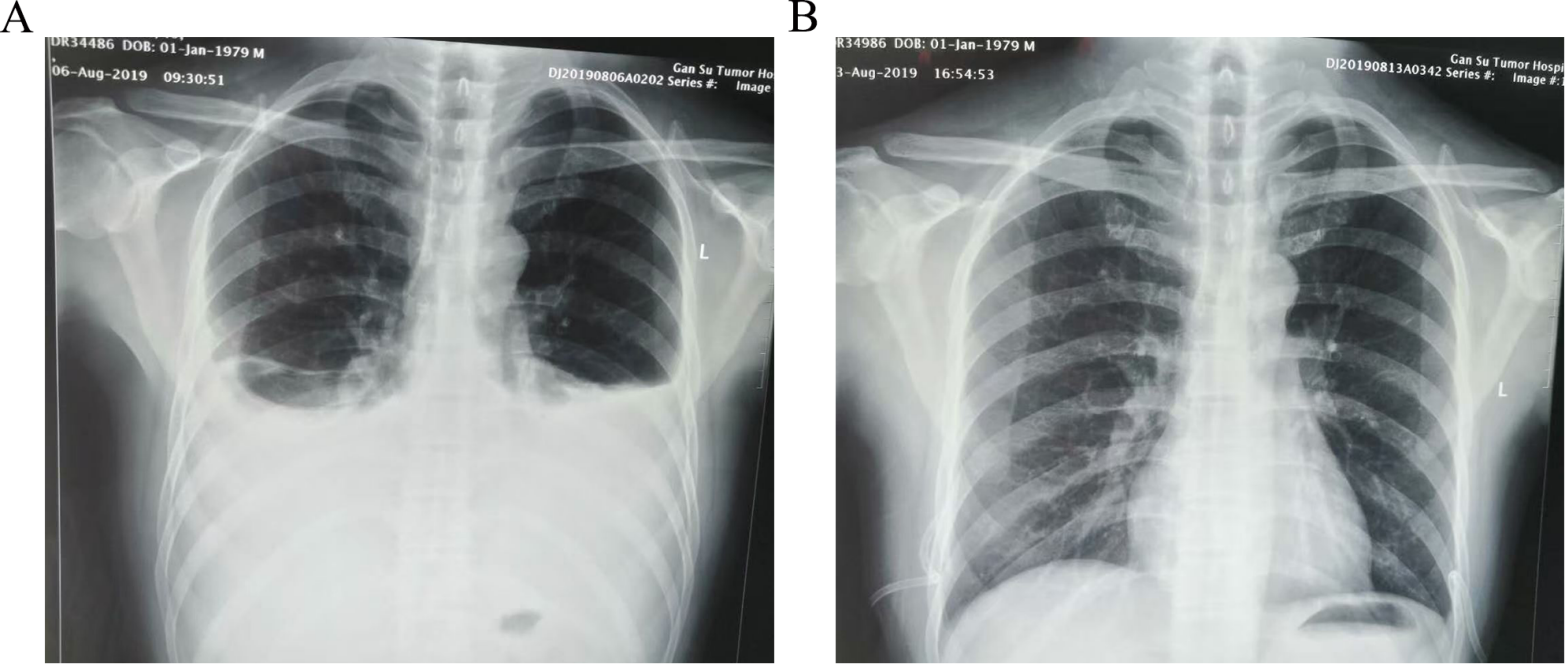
Chest X-ray images of patient before and after treatment (A):a chest radiograph revealed bilateral pleural effusion on postoperative day 7. (B) No obvious thoracic fluid collections in the review X-ray image on postoperative day 14.
